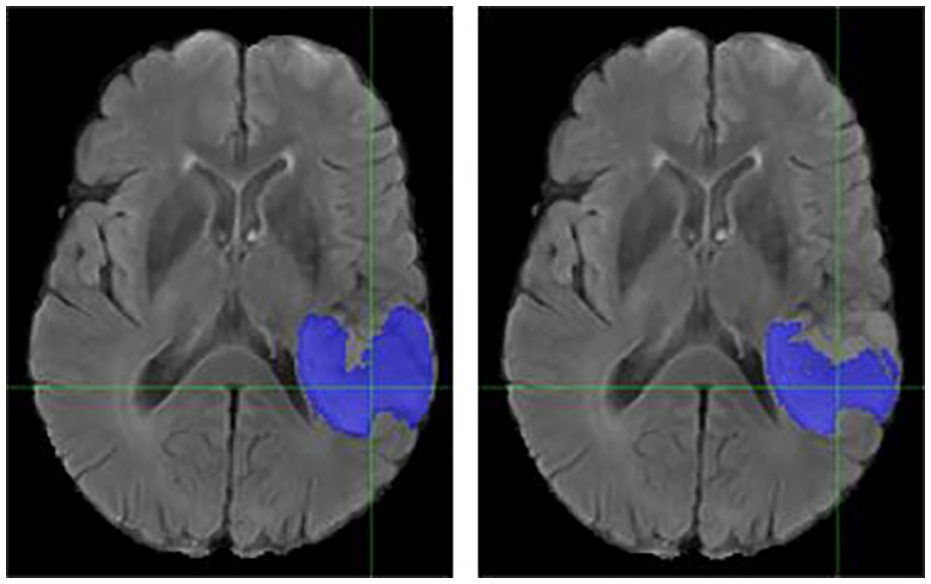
Case Two (Worsened). Low-Grade Glioma. Flair MRI. rMR only (Left) and Total SynMR MRI (Right) DSC of WT showed a decrease from 0.86 to 0.59.
